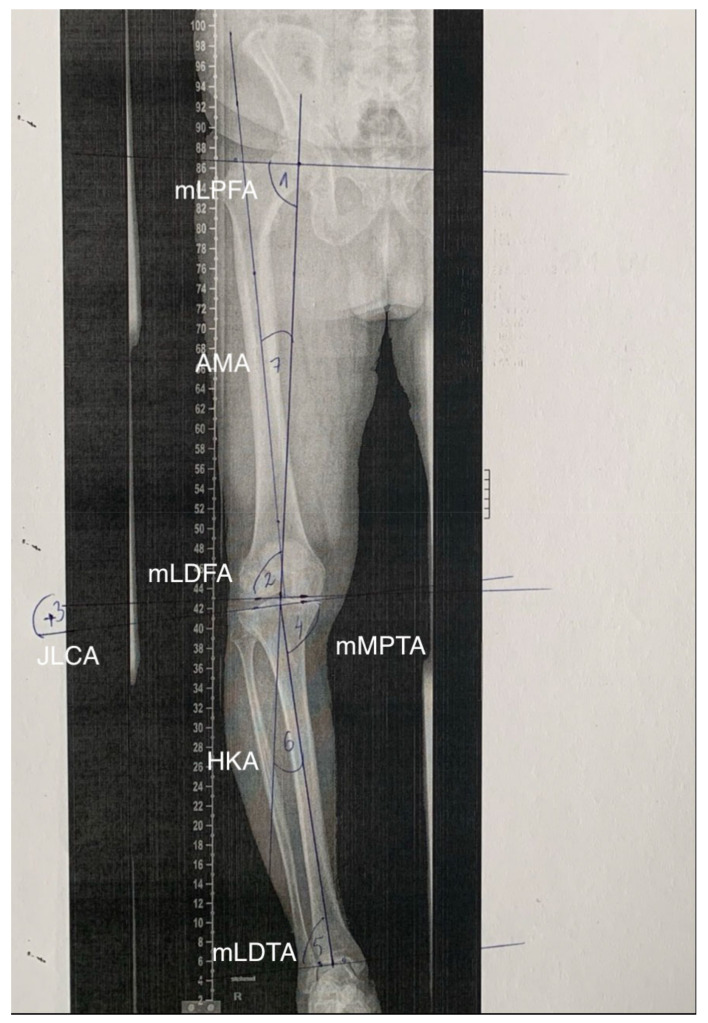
A graphical depiction of the angles measured on a long-leg radiographic view. (1) mLPFA; (2) mLDFA; (3) JLCA; (4) mMPTA; (5) mLDTA; (6) HKA; (7) AMA.
